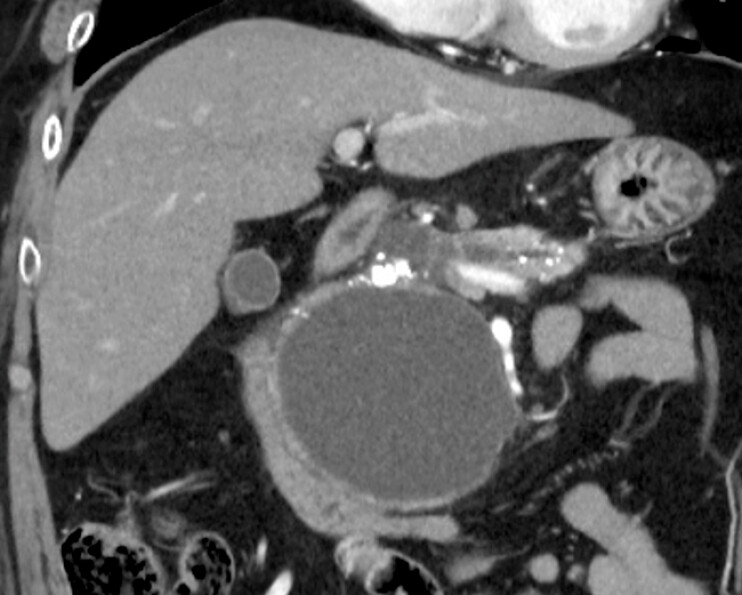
Computed tomography showing a 9-cm pseudocyst in the pancreatic head.
